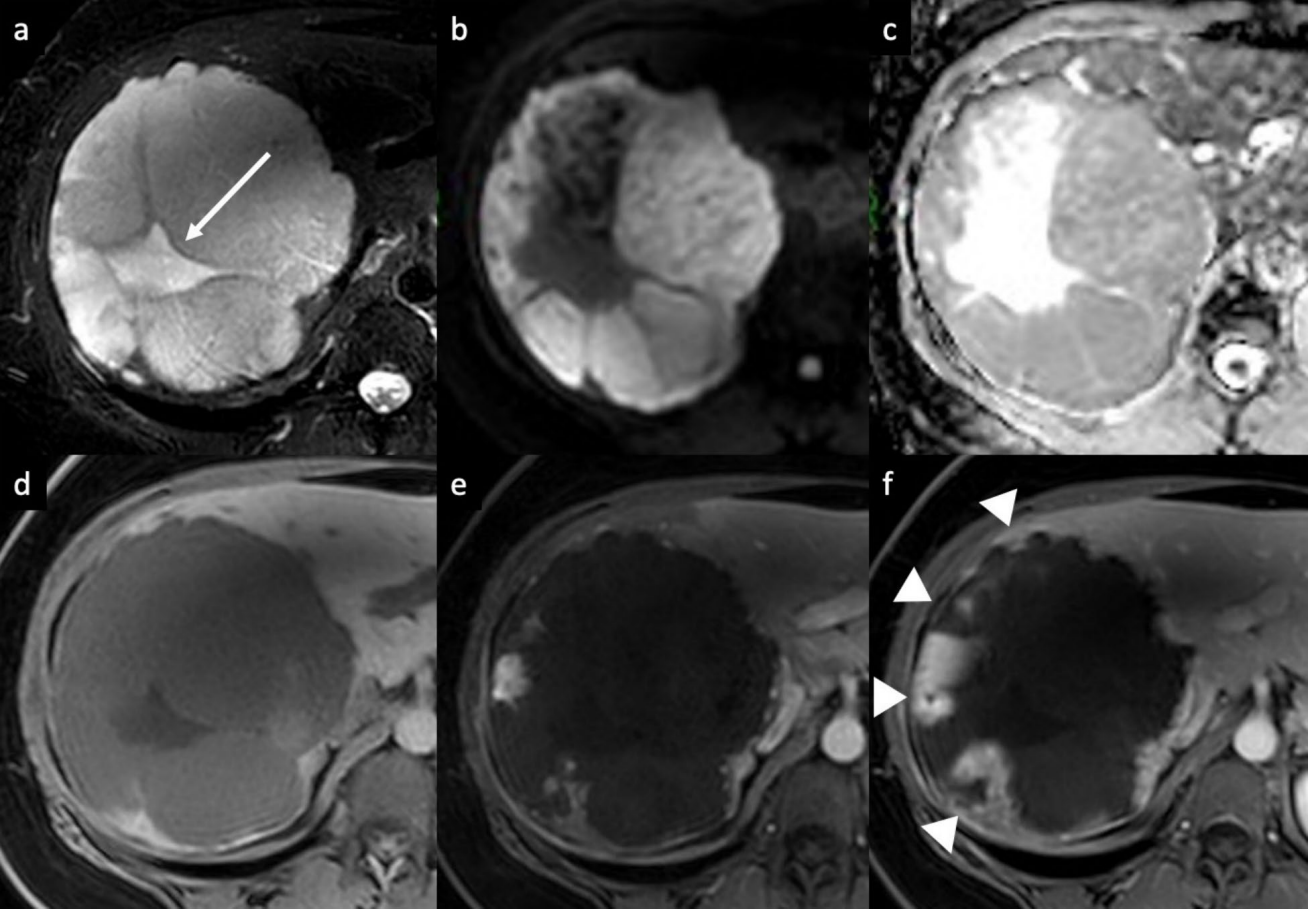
Giant hemangioma in a 40-year-old man who presented with abdominal pain. a Axial fat-suppressed T2-weighted MRI shows a large, lobulated, markedly hyperintense liver lesion located in the right hepatic lobe, with high signal intensity cleftlike area (arrow) and some hypointense internal septa. The lesion shows high signal intensity on diffusion-weighted images (DWI) (b) and high signal intensity on apparent diffusion coefficient (ADC) map (c); the cleftlike area and the internal septa appear hypointense on DWI, with marked high signal intensity on ADC map. The lesion displays a low signal intensity on unenhanced T1-weighted image (d), with peripheral discontinuous nodular enhancement on arterial phase (e), and progressive but incomplete peripheral filling on delayed phase (arrowheads, f)
